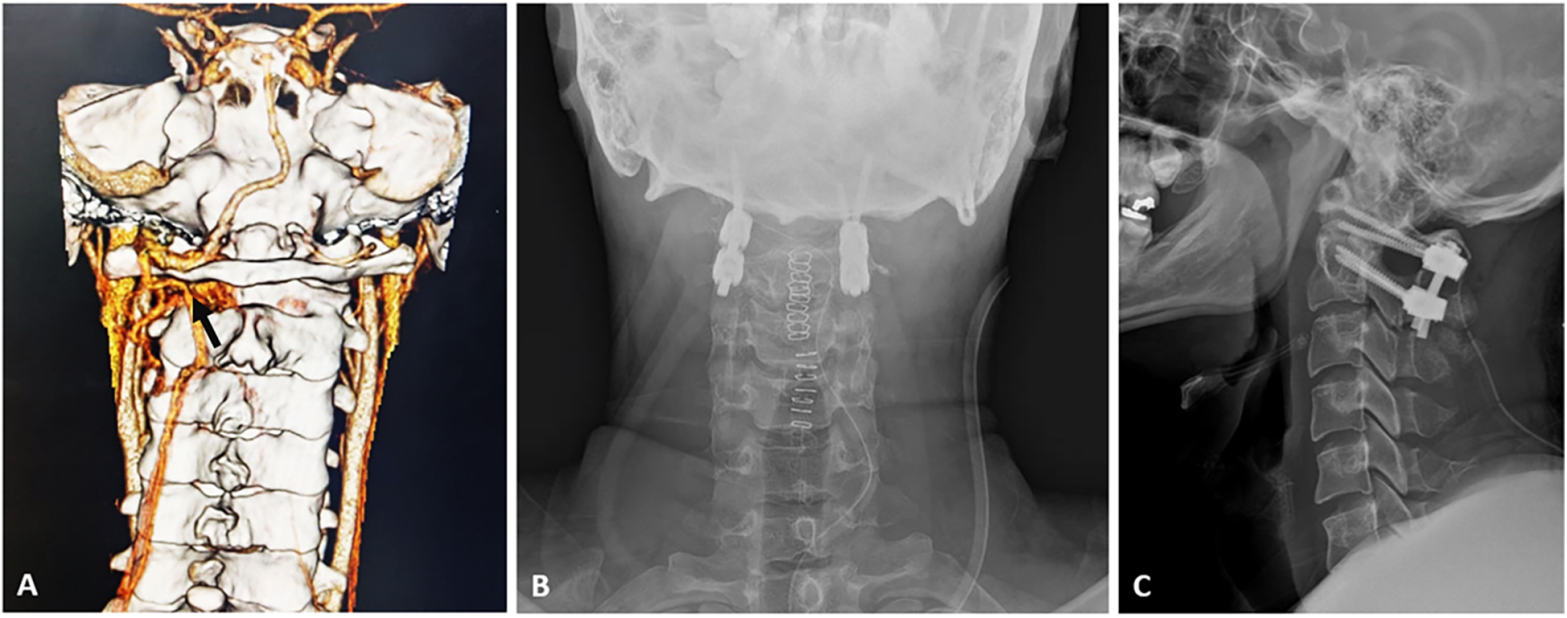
(A) Computed tomogram-angiography of Cervical spine showing abnormal course of left vertebral artery with tortuous and dilated vascular channels (black arrow) at C1–C2 level; post-operative antero-posterior (B) and lateral (C) radiograph showing a stable Goel-Harms construct under navigation guidance.
